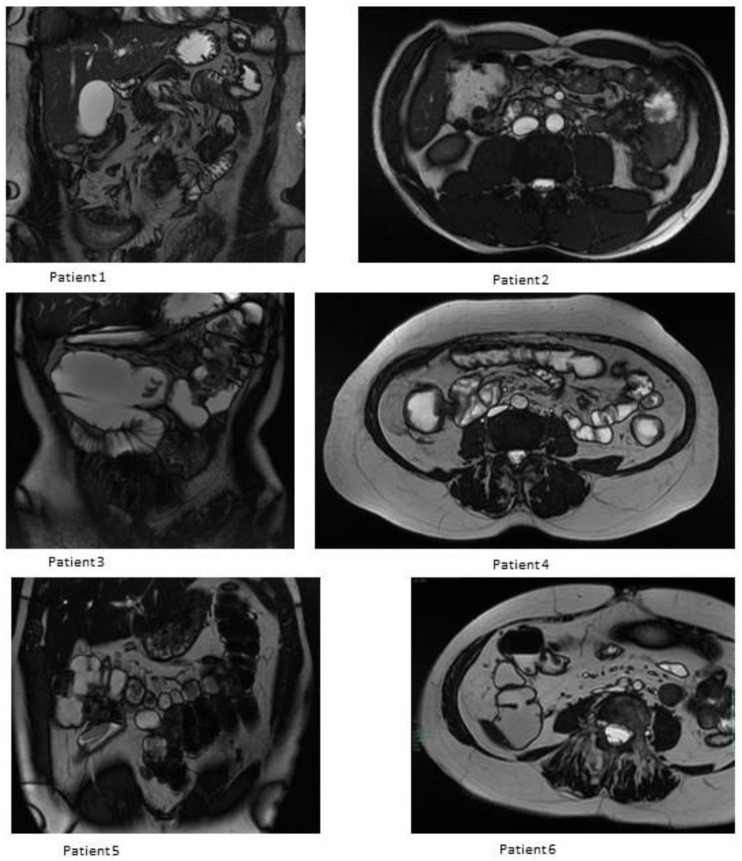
Anastomosis evaluation by magnetic resonance imaging after the first year of follow-up.
